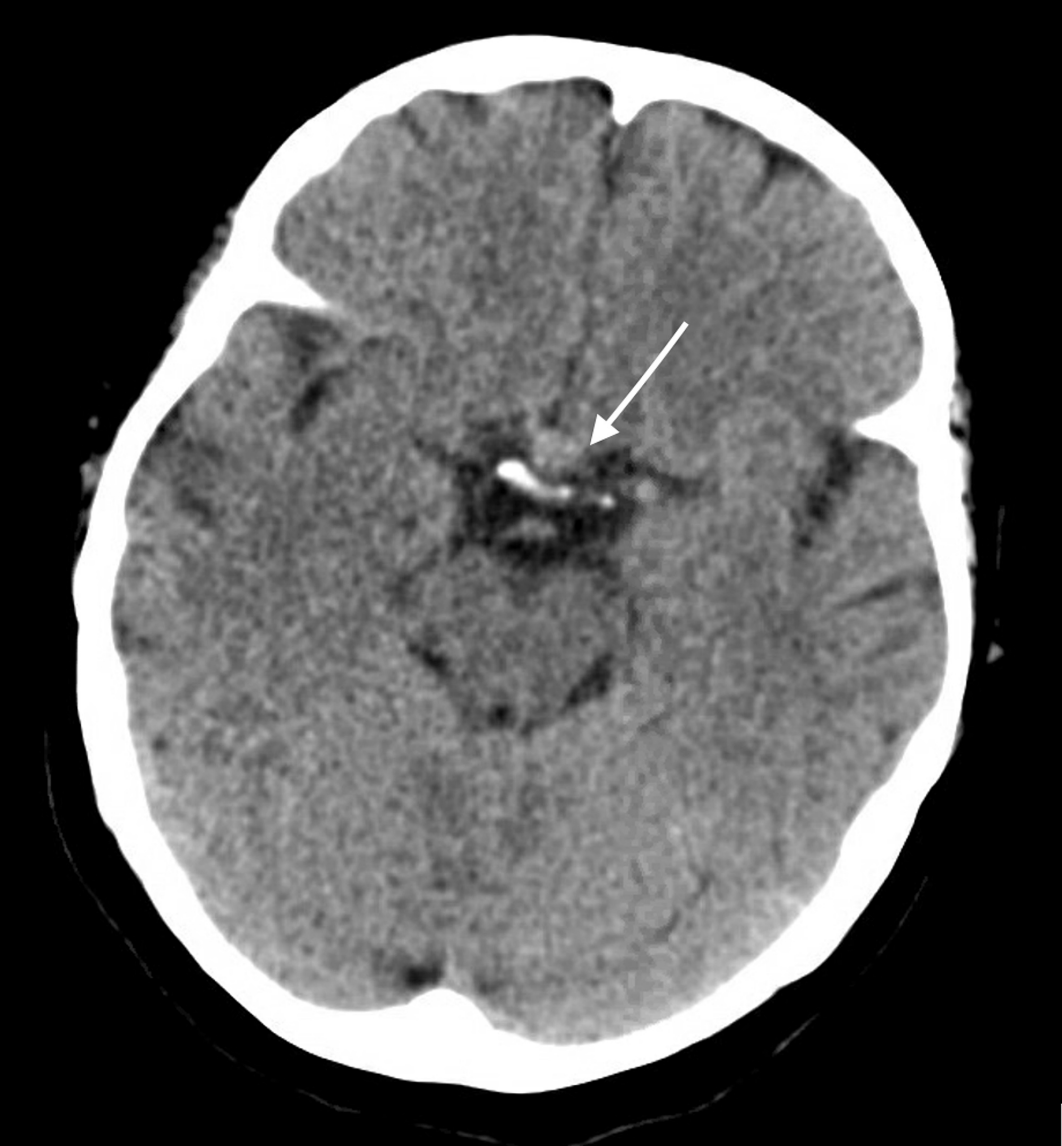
Axial unenhanced CT scan of the brain demonstrates an 8 mm sellar/suprasellar high-density lesion with mass effect on the optic chiasm (arrow) CT, computed tomography
